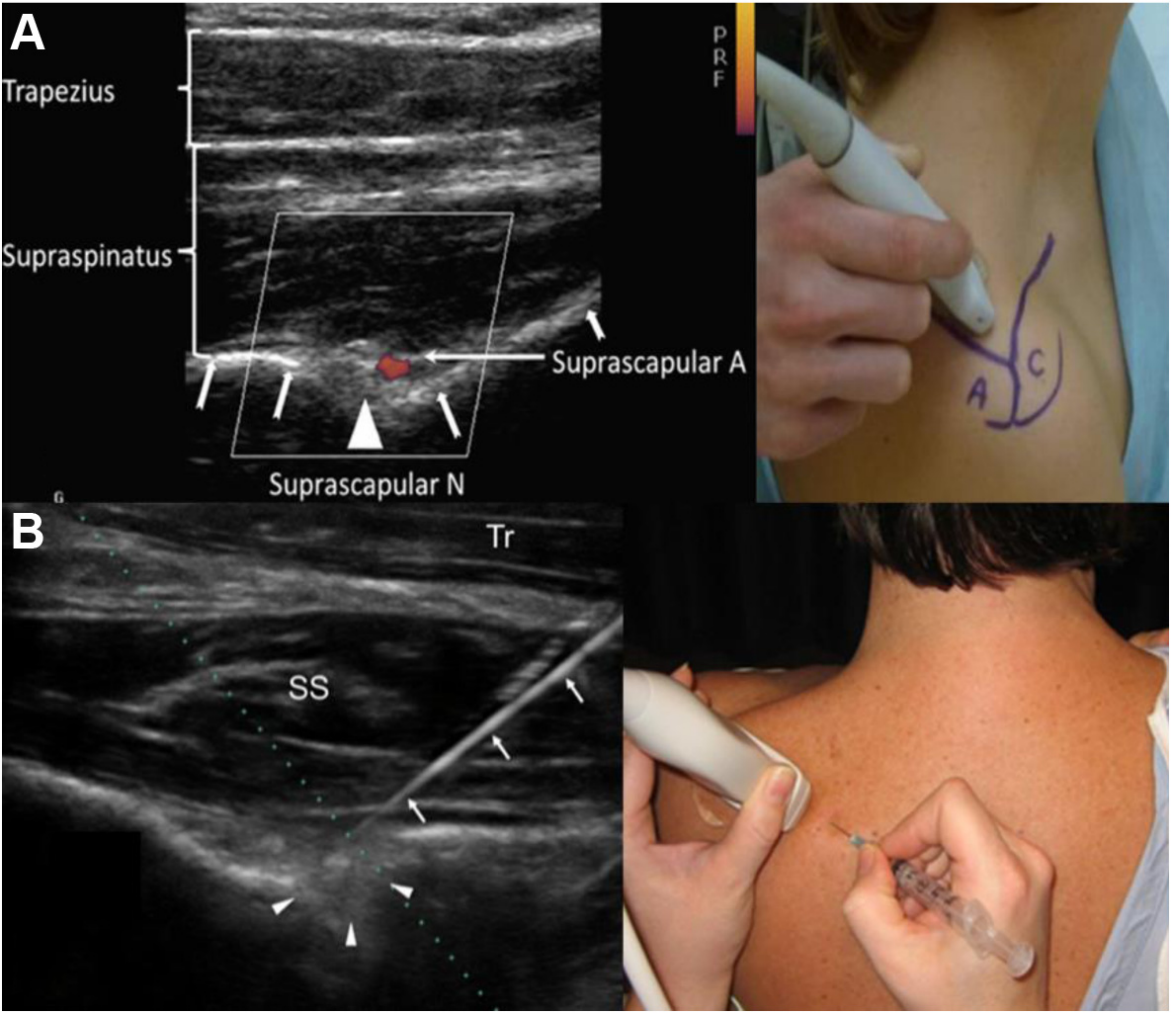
Ultrasound-guided injection of local anesthetic targeted to the suprascapular notch, demonstrating 2 different injection techniques. (A) Superior approach, with additional Doppler utilization to identify the suprascapular artery (*white arrows* with split tails demonstrate needle path and infiltration of anesthetic fluid). (B) Posterior approach. *SS*, supraspinatus; *Tr*, trapezius; *white arrows*, needle; *white arrowheads*, infiltration of fluid in the suprascapular notch.
